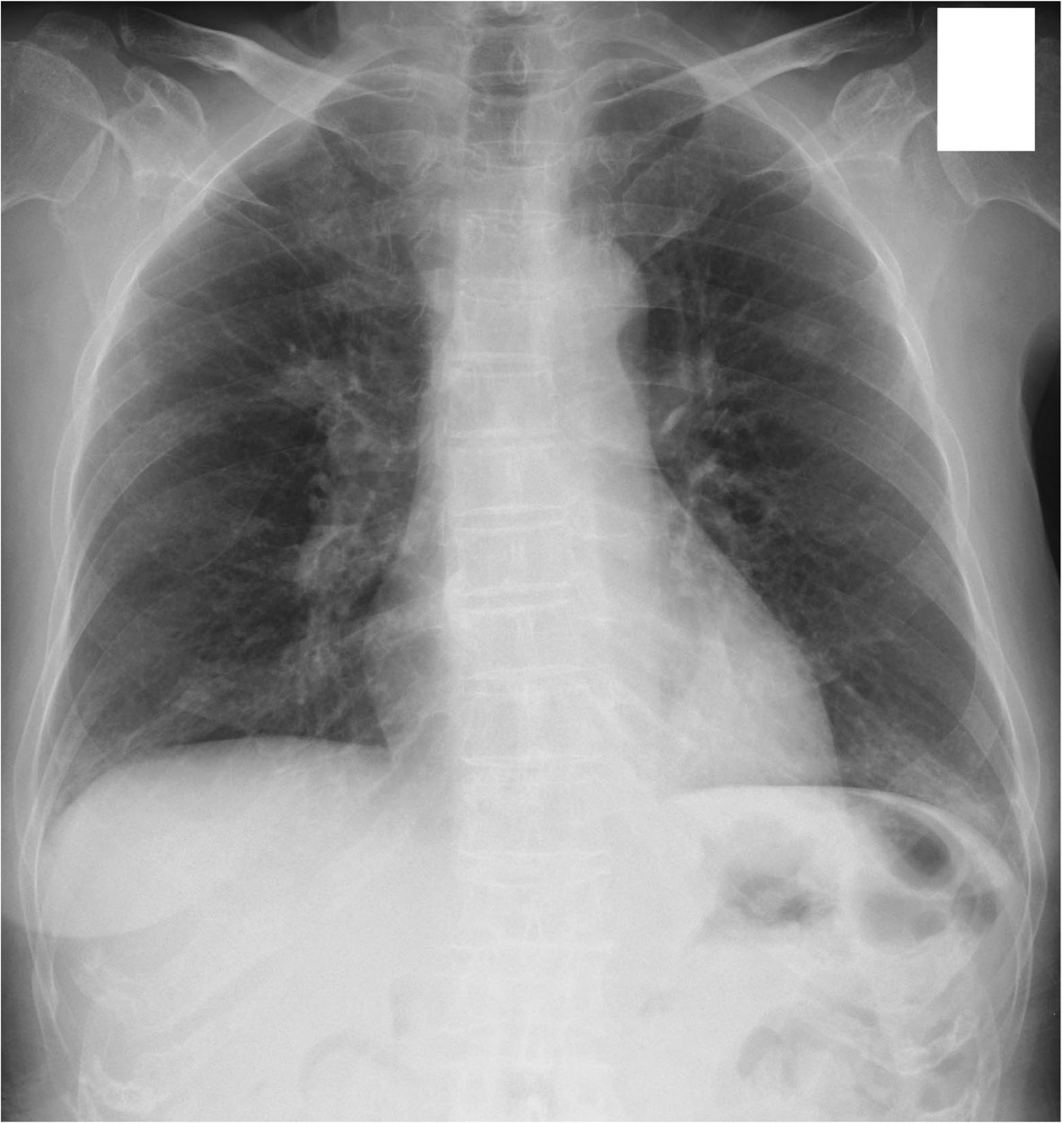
Chest radiograph obtained prior to the second spinal fusion showing no pleural effusion
